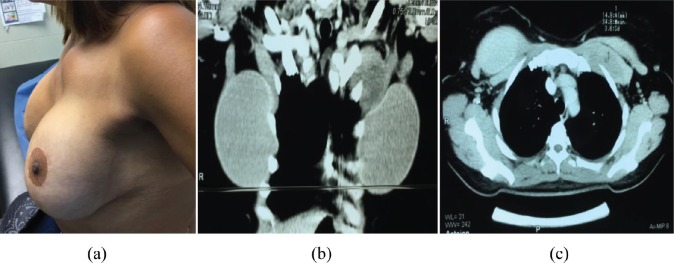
Fourth case. (a) Patient with fibromatosis of left breast at initial physical exam: tumour of left breast. (b) CT of the thorax, coronal incision. (c) CT of the thorax, axial incision: heterogeneous lesion retropectorally located.
